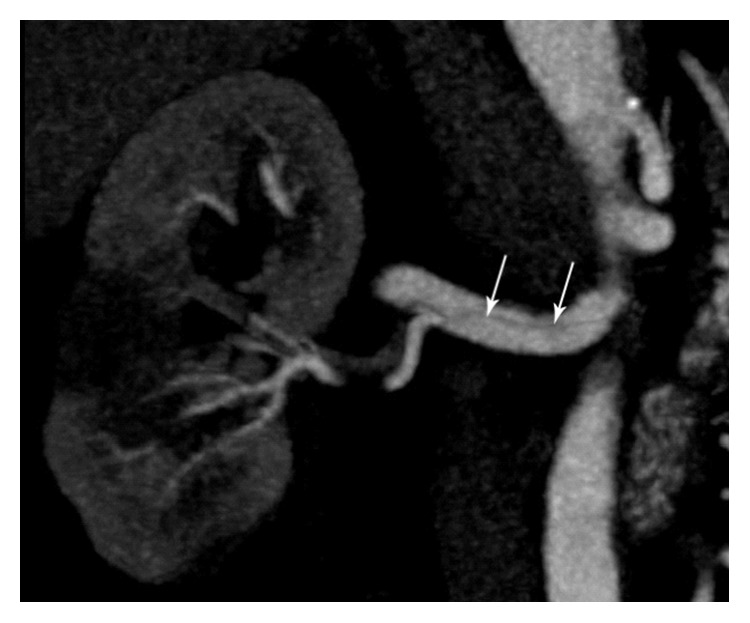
Findings: maximum intensity projection, in coronal plane of CT renal angiography in arterial phase demonstrating linear filling defect in main renal artery, suggests intimal flap (long arrow).
